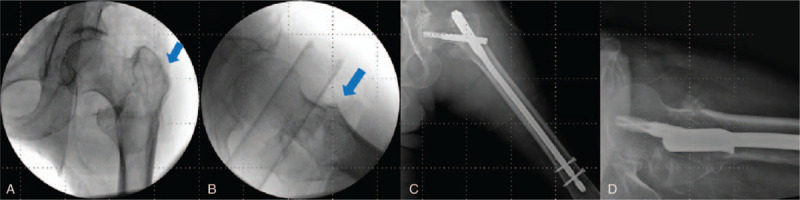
Case 1. (A) and (B) Intraoperative C-arm images showed proper traction and maintained reduction (blue arrow). (C) Postoperative radiographs showing adequate fracture reduction and fixation. (D) True lateral radiographs showed an apposition of the anterior cortex.
